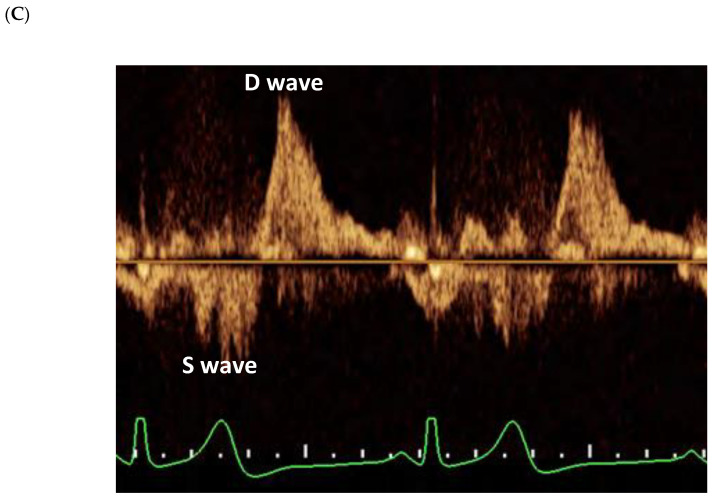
Pulmonary Venous Flow Patterns as Imaged on Transesophageal Echocardiogram. Normal pattern is signified by a peak systolic (S) to peak diastolic (D) flow velocities ratio of ≥1. Abnormal pattern may include either a blunted or a reversed S wave, manifested by an S/D ratio of <1 or <0, respectively. (A) Normal Flow Pattern. (B) Blunted Flow Pattern. (C) Reversed Flow Pattern.
